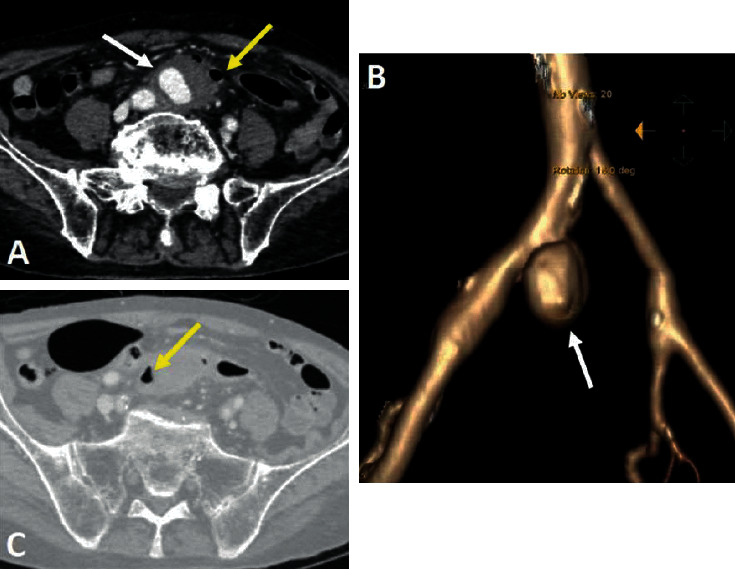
CT scanner at the time of admission. (a, b) Enhanced axial and reconstruction coronal CT shows a 31 × 36 × 40 mm saccular aneurysm (arrows) arising from the right common iliac artery and prominent inflammatory soft tissue around the aneurysm. The anterior wall of the aneurysm has adhered to the posterior wall of the sigmoid colon ((a), yellow arrow). Gas foci ((c), yellow arrow) were noted around the aneurysm.
